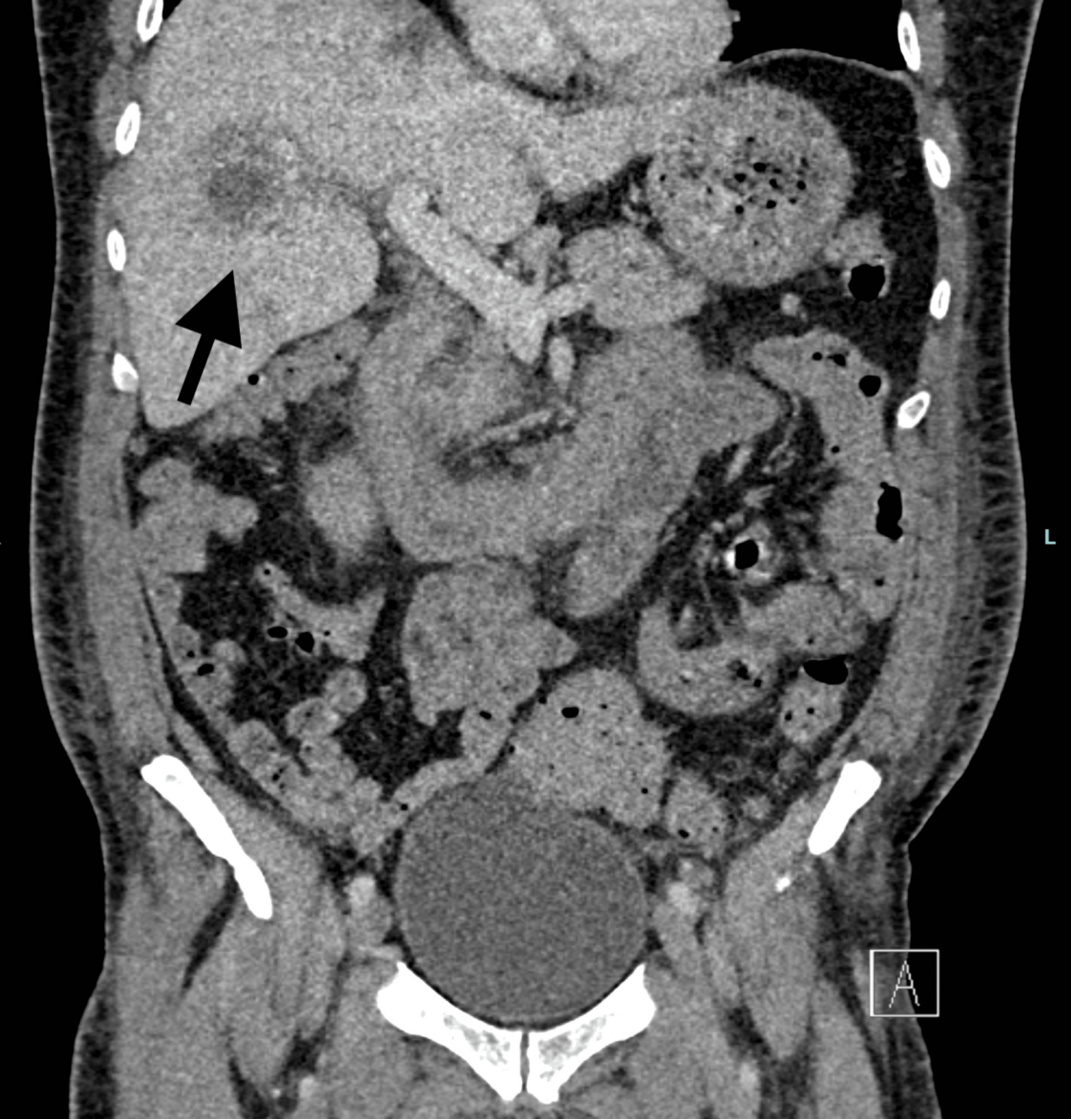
Contrast-enhanced CT abdomen demonstrating multiple low-attenuation lesions within the right hepatic lobe, consistent with evolving hepatic abscesses. The distribution and appearance support portal venous spread from an intra-abdominal source, in keeping with polymicrobial sepsis.
